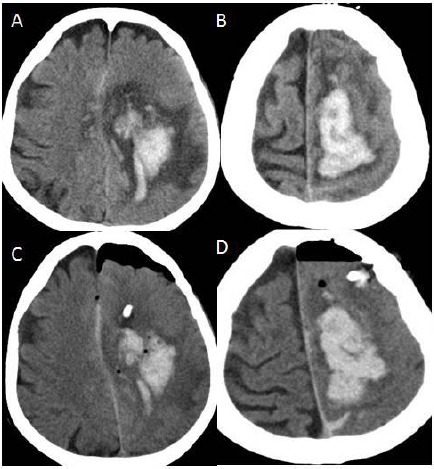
A, B: Cranial CT of the axial sections showing hematoma at the left centrum semiovale after biopsy procedure; C, D: After hematoma evacuation*.
